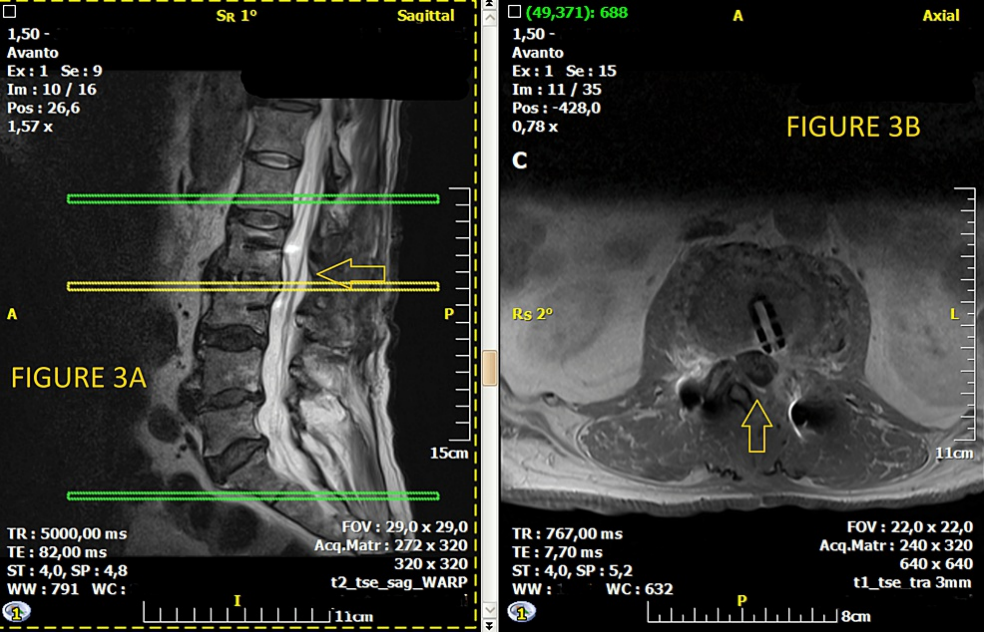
(A) At the left sagittal postoperative view of MRI scan; (B) at the right axial postoperative view of MRI scan MRI scan of the lumbar spine with no evidence of significant spinal cord compression
